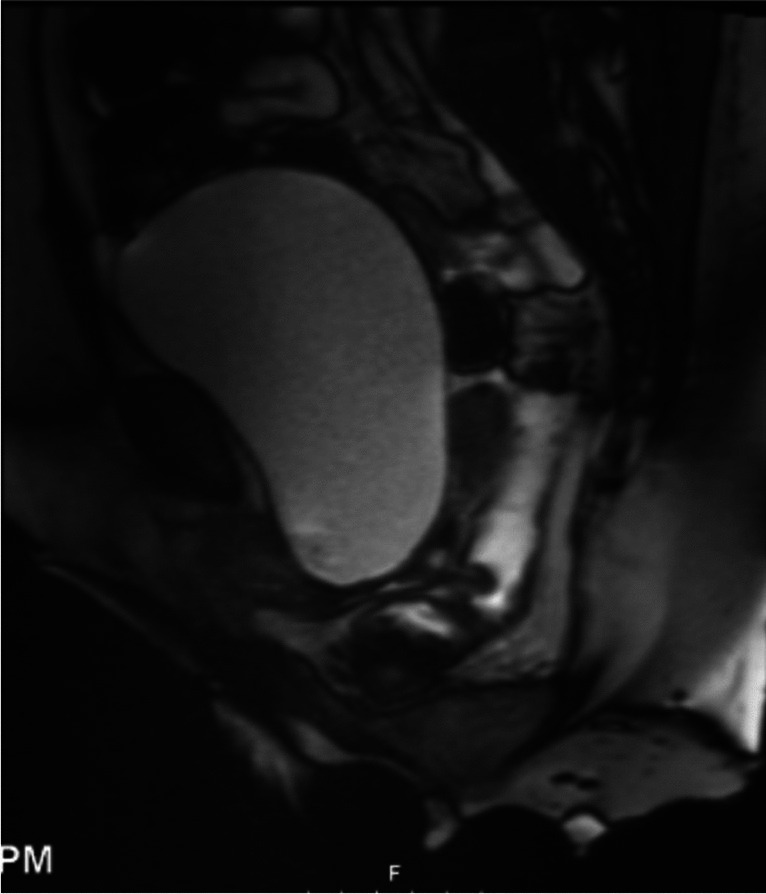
Magnetic resonance image demonstrating a posterior defect with enterocele containing small bowel and small bowel mesentery
